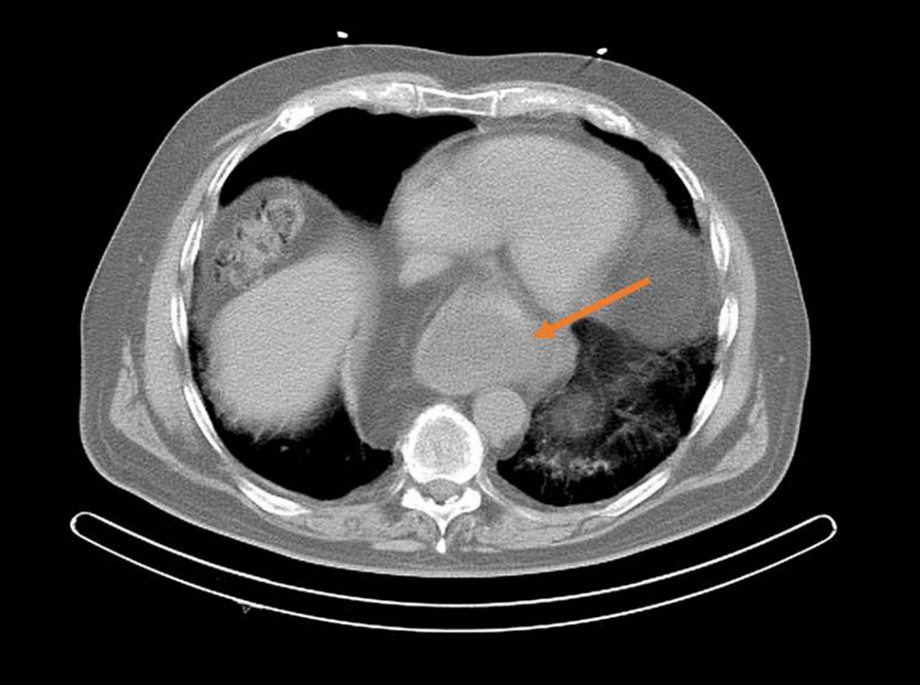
Image of computed tomography made for the purposes of attenuation correction during SPECT/CT. Suspicion of large sliding hernia of the diaphragm was raised (red arrow)
